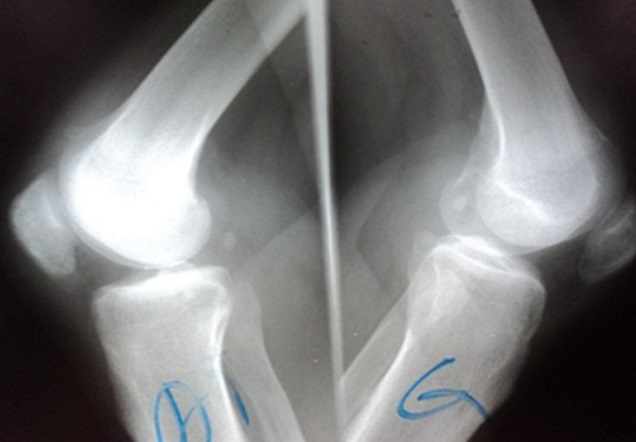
Lateral radiographs showing suprapatellar calcifications
